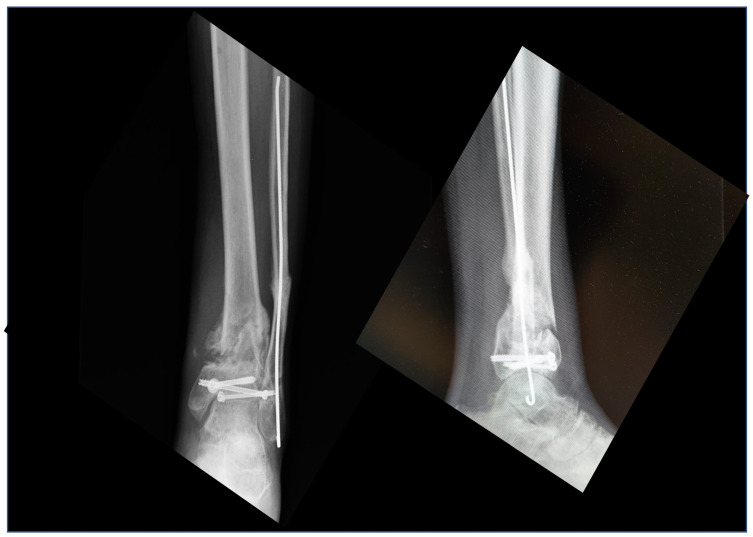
Pre-operative X-rays showing a case of septic non-union of the tibial pilon.
